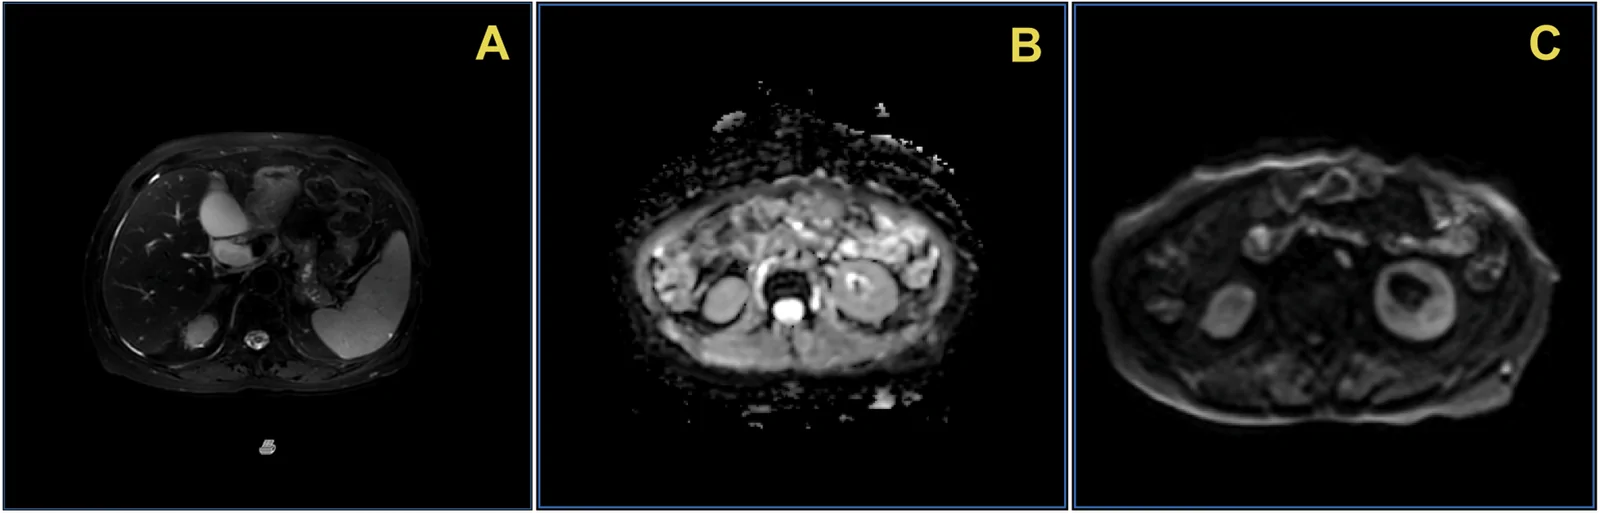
MRI showing gallstones, common bile duct stones, biliary obstruction, and a mass at the duodenal papilla. (A) Gallbladder stones and bile duct stones. (B) ADC shows low signal (restricted diffusion); and (C) DWI shows high signal at the papilla; suggesting mass at the duodenal papilla.
